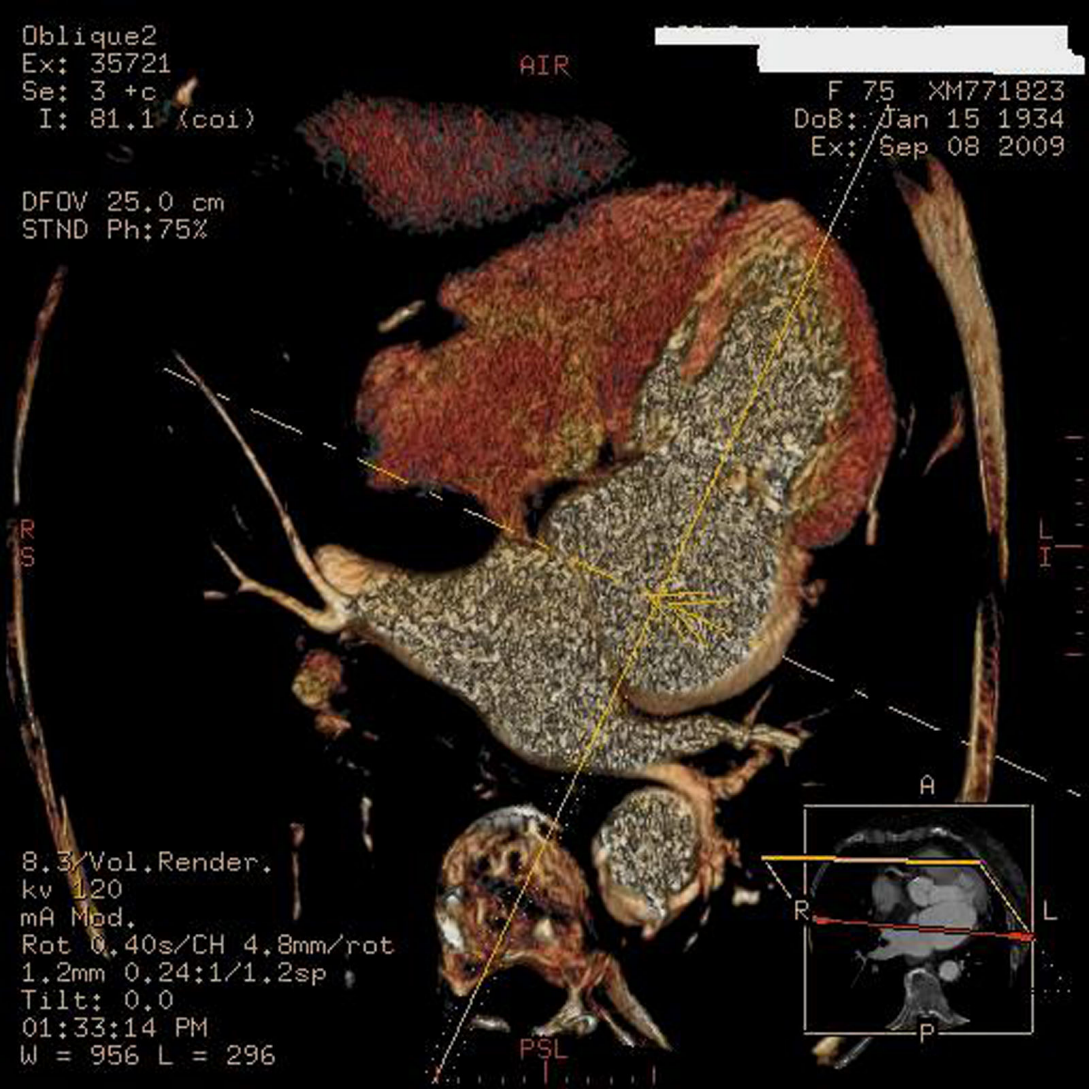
Three dimensional reconstruction of computed tomography shows the fibromuscular membrane dividing the left atrium into two distinct chambers.
